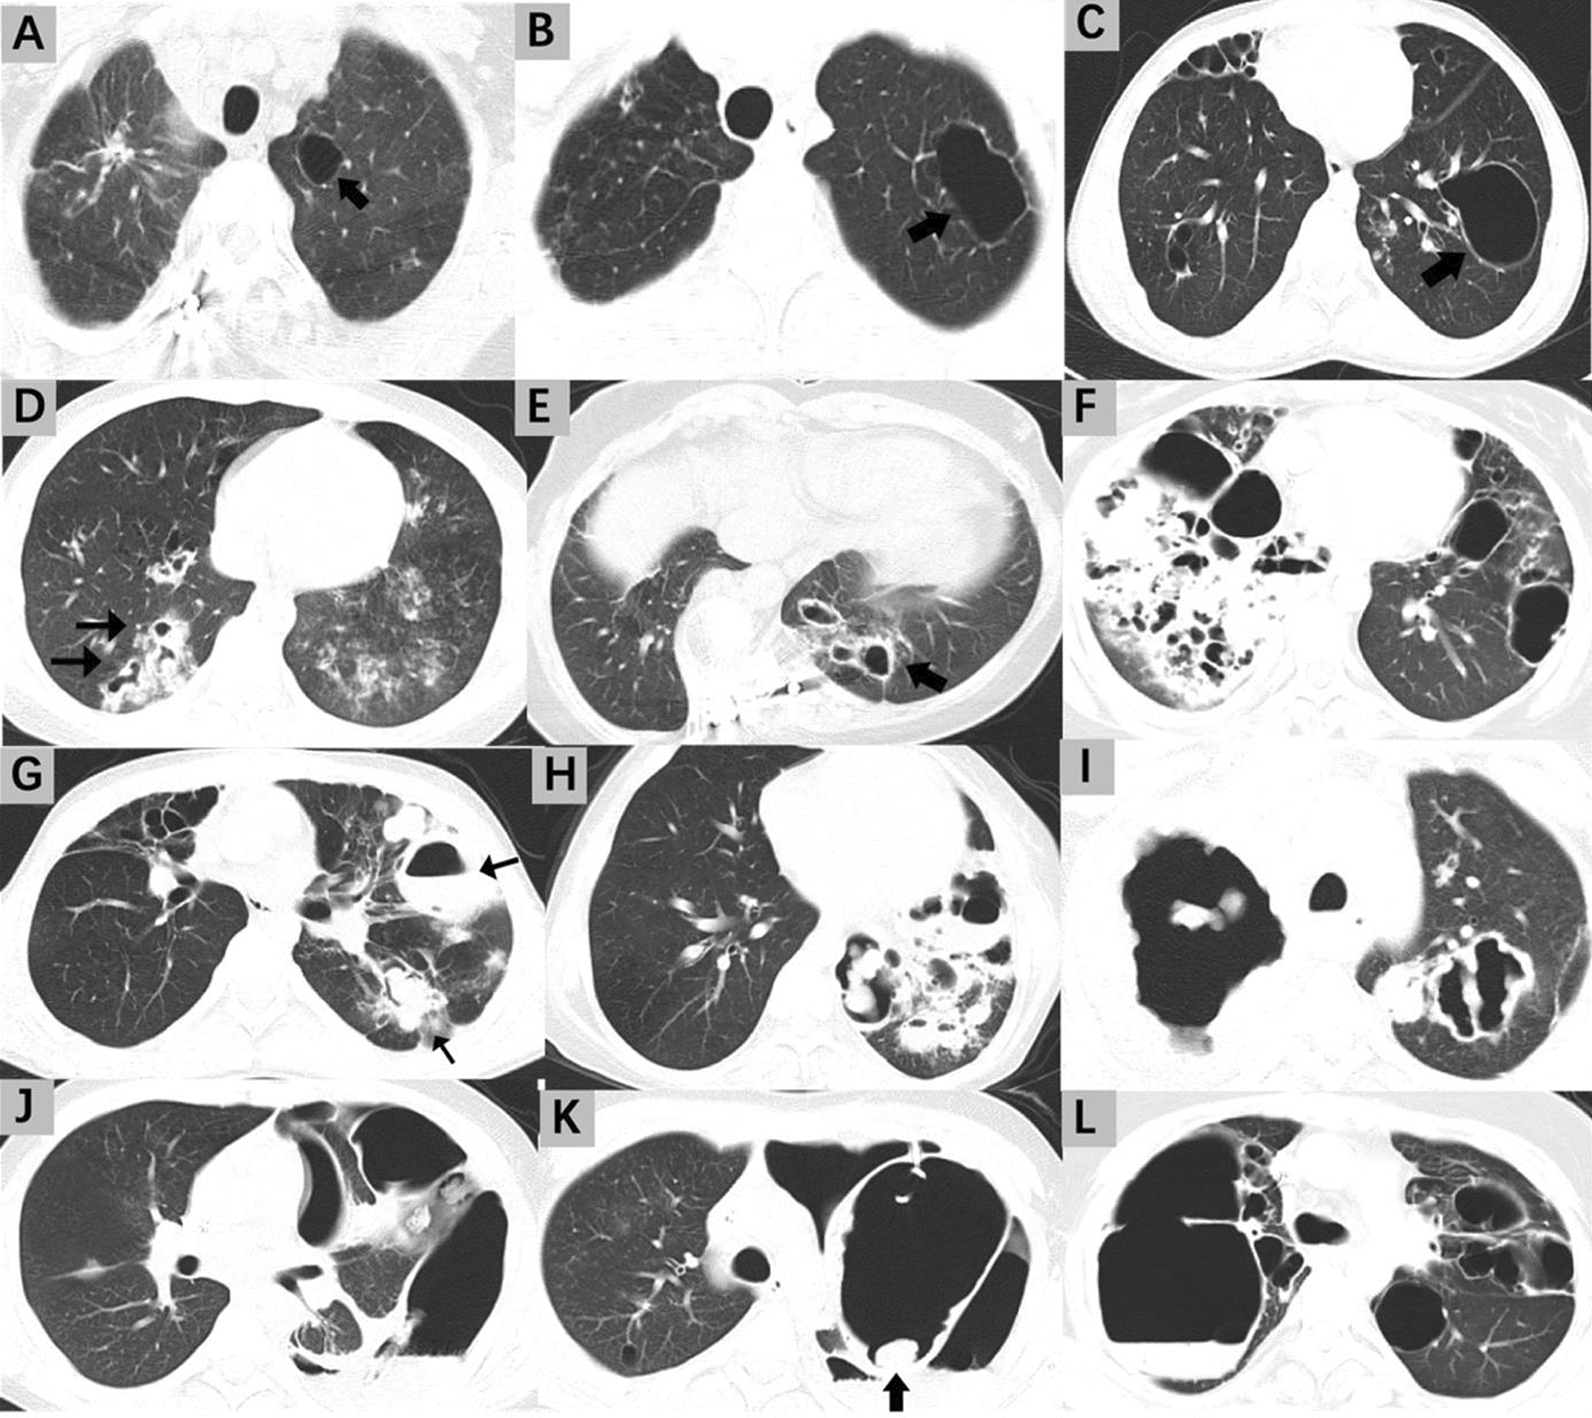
Chest CT of characteristic lung damage of parenchyma and airways in adult patients with STAT3-HIES. A (Patient #6): Thin-walled cysts in the left upper lobe. B&C (Patient #4): Large pneumatoceles in the left upper lobe. D (Patient #7): Ground-glass opacities and marked bronchiectasis with mucoid impactions. E (Patient #6): Central bronchiectasis and visible dilatation of the airways. F (Patient #5): Multiple cavitary lesions with thickened walls, marked bronchiectasis, and surrounding consolidation, indicative of CCPA. G (Patient #4): Bronchiectasis with a finger-in-glove sign, indicating bronchial impaction with mucus and air-fluid levels, suggestive of ABPA. H (Patient #9): Cavitary lesions with thick posterior walls and air-fluid levels. I (Patient #8): Extensive cavitation with thick posterior walls. J (Patient #7): Pneumothorax with mediastinal shift, secondary to a large ruptured pneumatocele or bulla. K (Patient #7): Giant bulla with significant compression of adjacent lung tissue; there is a centrally located aspergilloma within one of the cavities. L (Patient #5): Multiple pneumatoceles and thick-walled cystic spaces, indicating severe structural lung damage
